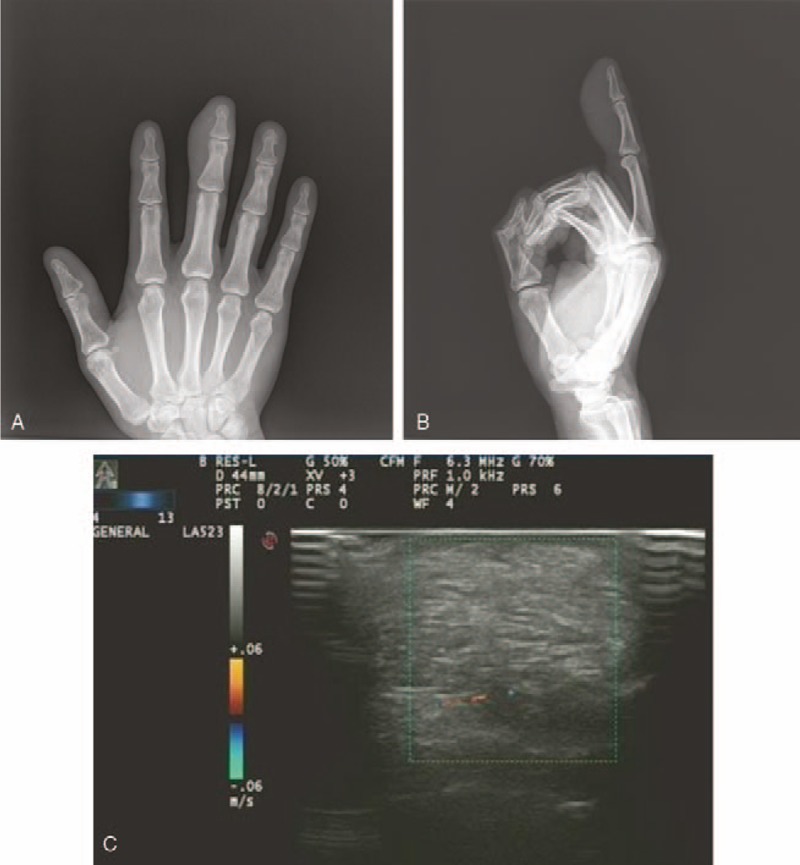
A: Radiography of the middle finger shows a soft tissue swelling of low density over the distal phalanx (anteroposterior view). B: Radiography of the middle finger shows a soft tissue swelling of low density over the distal phalanx (lateral view). C: An ultrasound scan shows a well-defined hyperechoic subcutaneous mass and no internal flow is detected.
